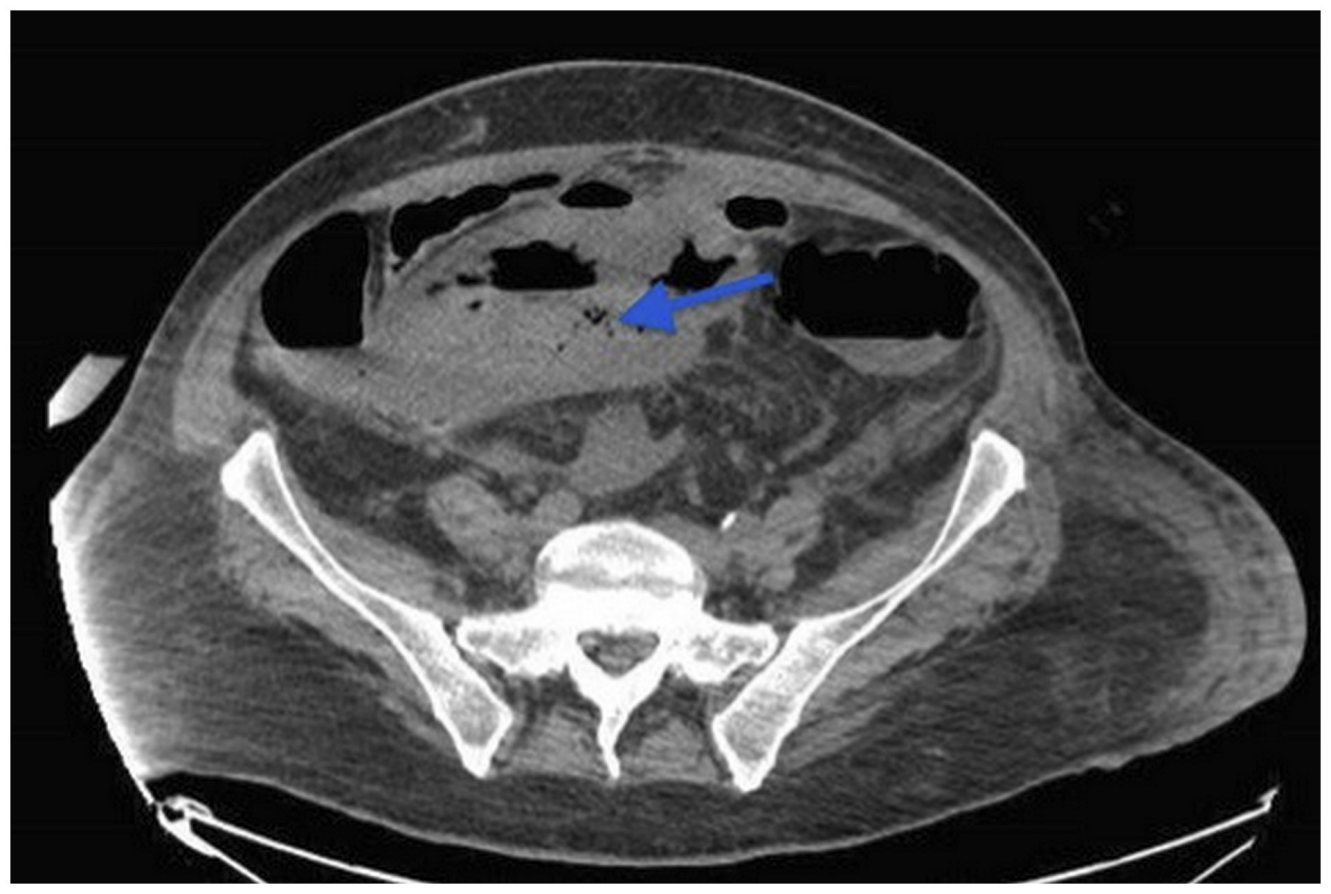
CT scan of the abdomen and pelvis revealed the presence of gas inside the pelvic mass (arrow) due to fistulisation of an intestinal loop with localized peritonitis.
